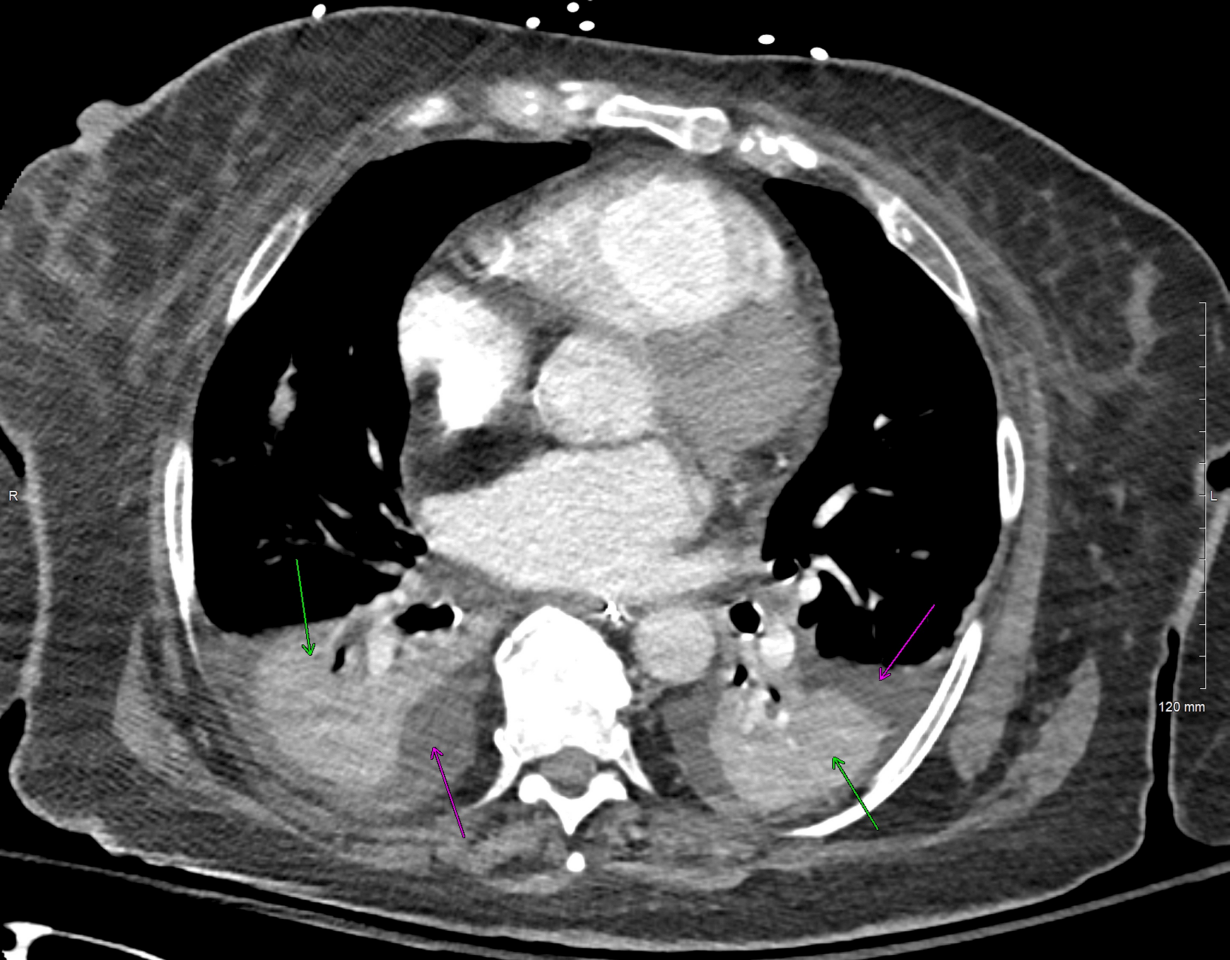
CT chest, axial slice, soft tissue window, showing bilateral pleural effusions (pink arrows), with associated complete collapse of the left lower lobe and near-complete collapse of the right lower lobe (green arrows)
